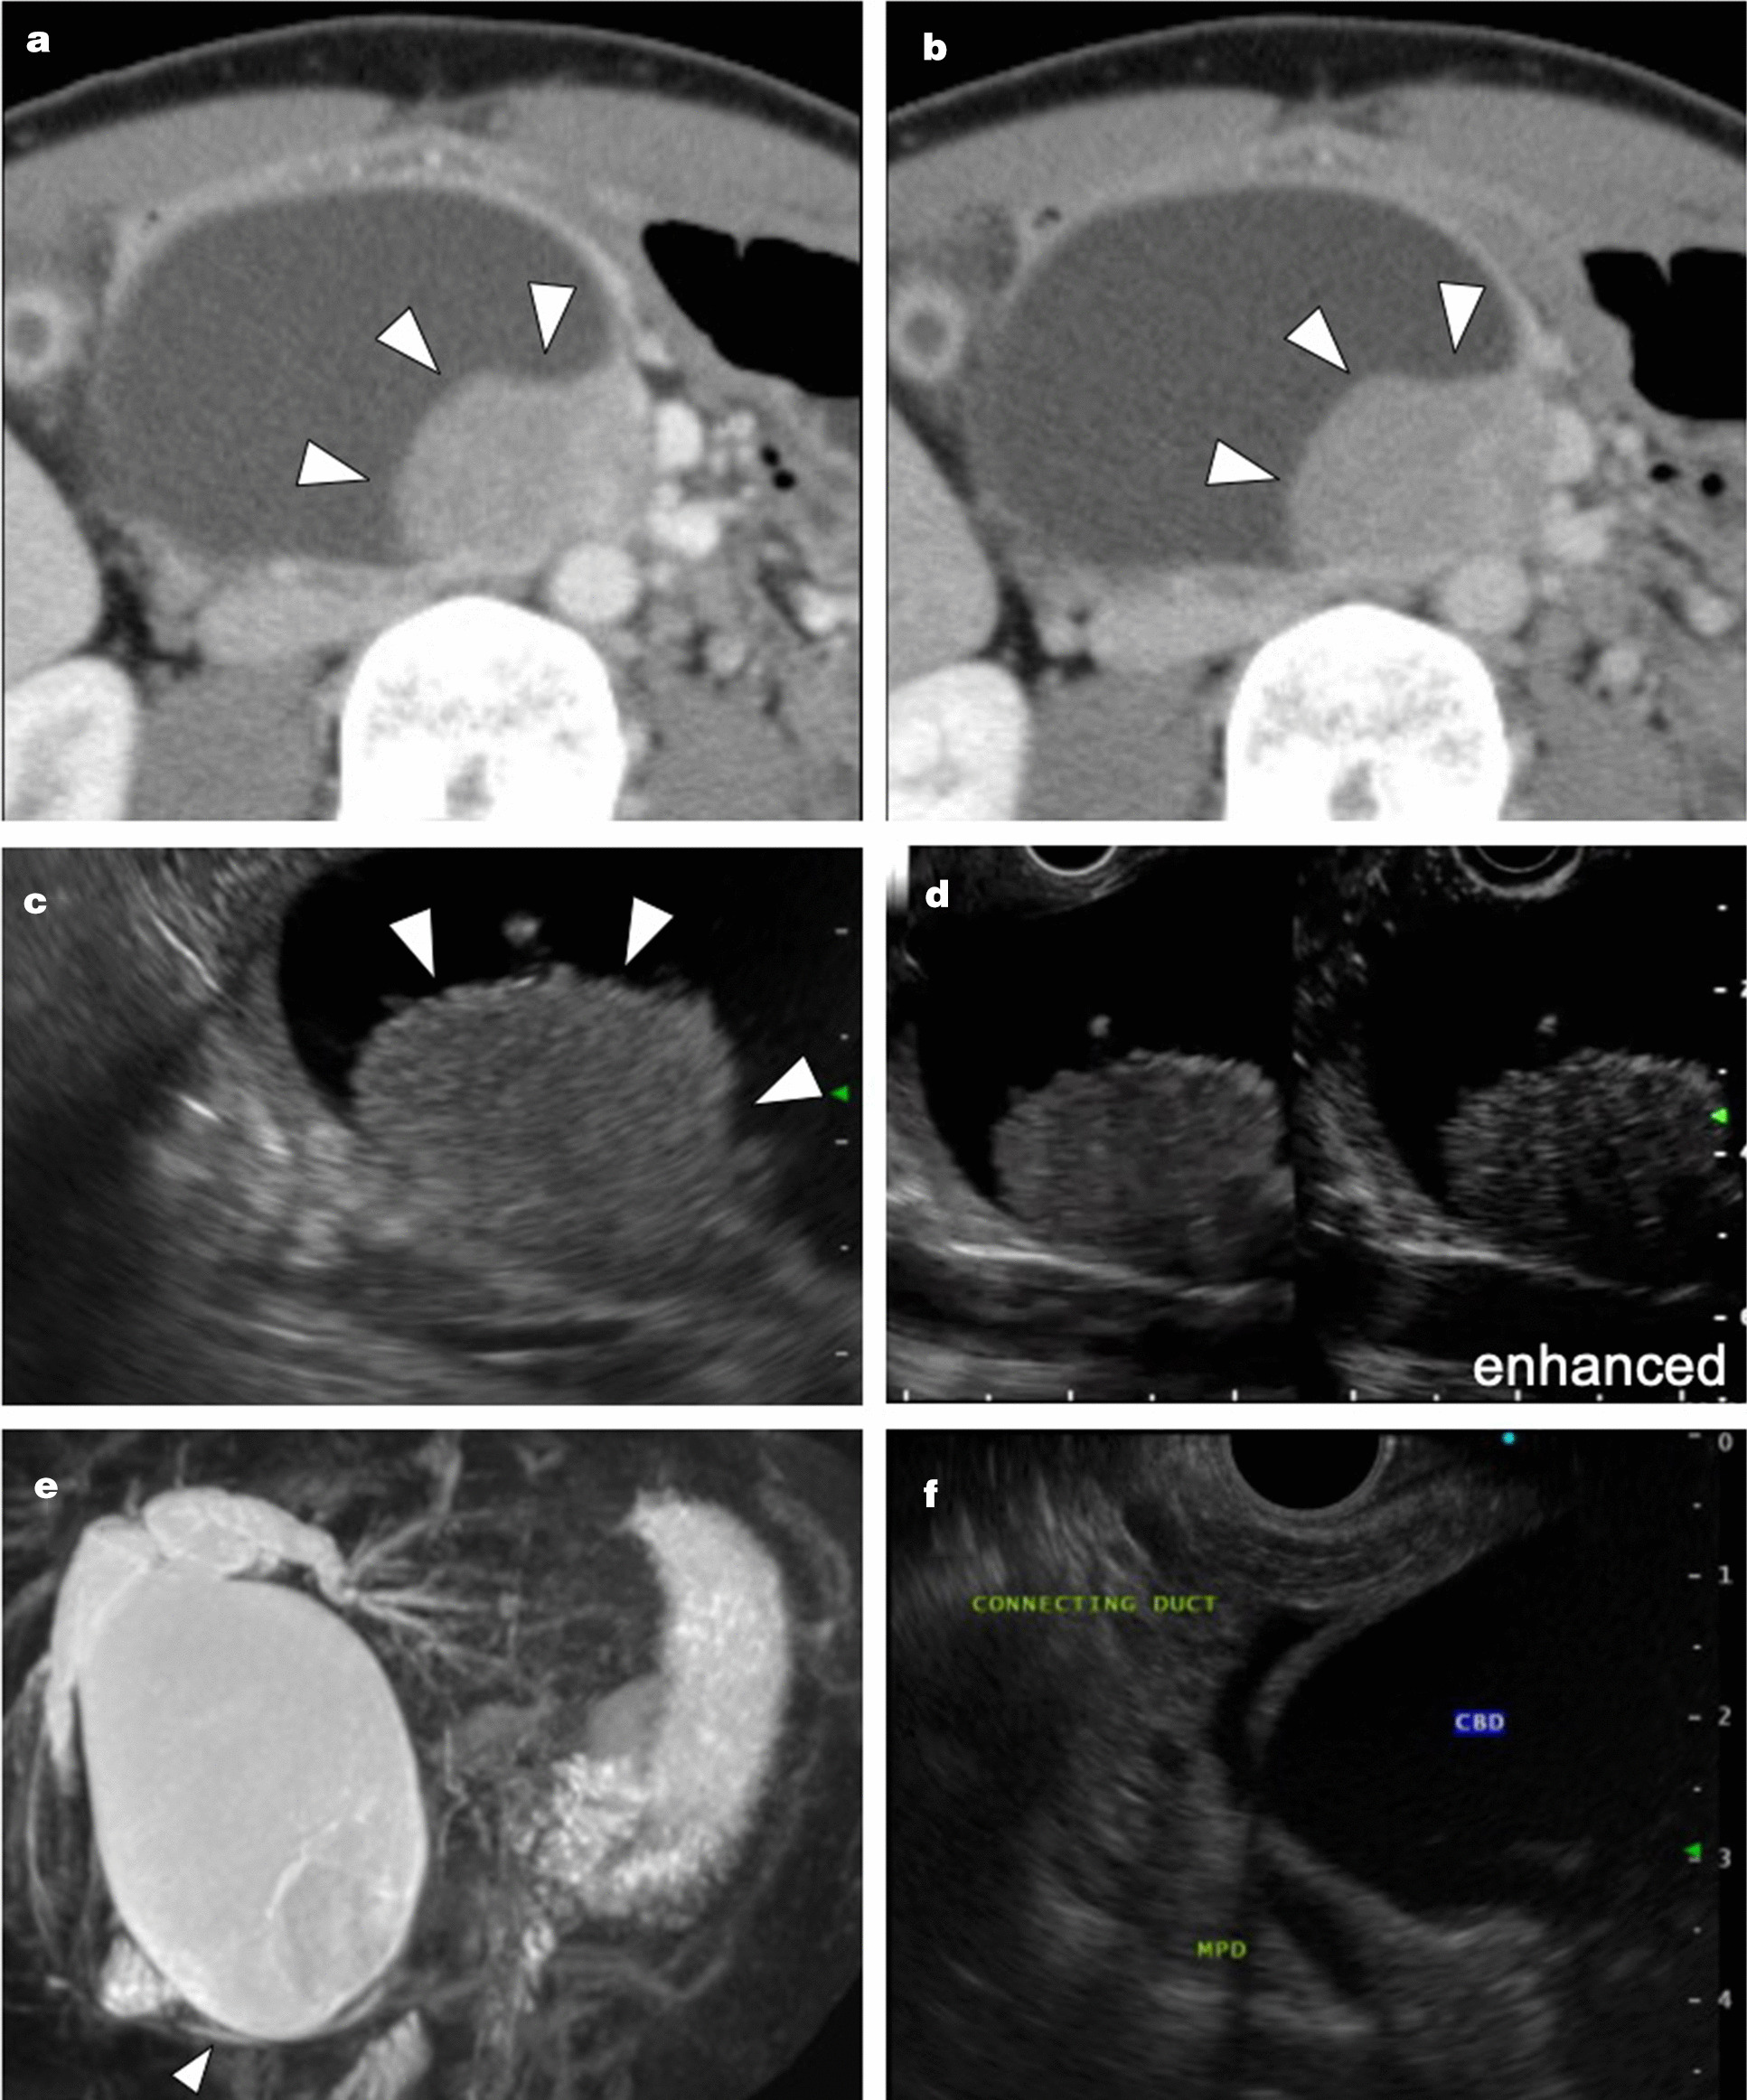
Preoperative imaging. a, b Arterial phase and delayed phase of abdominal dynamic contrast-enhanced computed tomography. These images show a tumor (arrowhead) in the middle portion of the common bile duct and dilatation of the common bile duct and the intrahepatic bile ducts bilaterally; the dilatation is diagnosed as Todani type IV-A. The tumor has enhanced iso-density at the arterial phase, and contrast effect was prolonged to the delayed phase. c Endoscopic ultrasonography shows a tumor (arrowhead) in the cystic dilated common bile duct. d Contrast-enhanced endoscopic ultrasonography shows the uptake of the contrast agent into the tumor. e, f Magnetic resonance cholangiopancreatography and endoscopic ultrasonography show one centimeter of the common channel (arrowhead), which continued from the duodenal papilla
